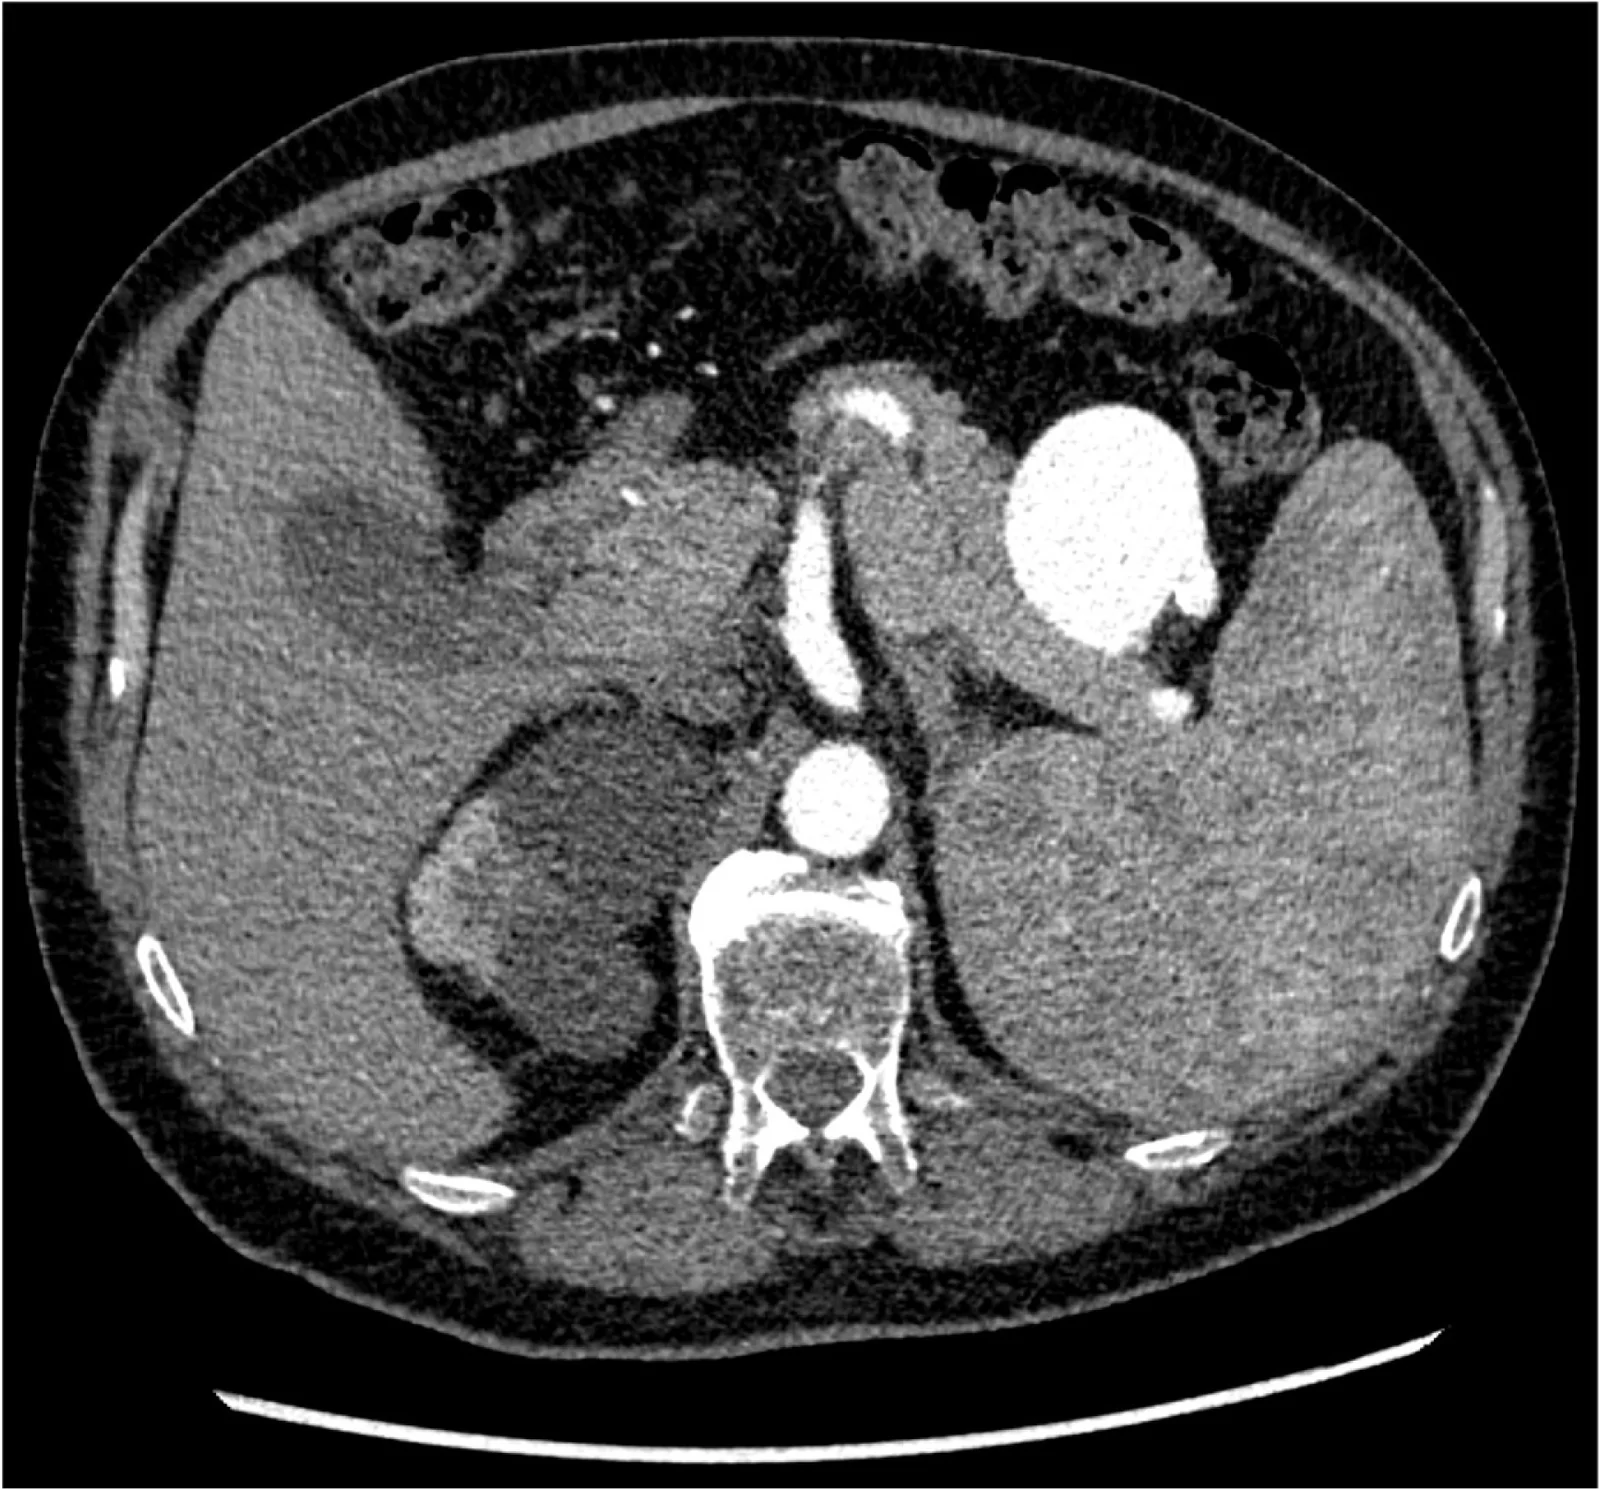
CT angiogram chest shows splenomegaly and partially imaged 4.3 cm splenic artery aneurysm within the splenic hilum.
